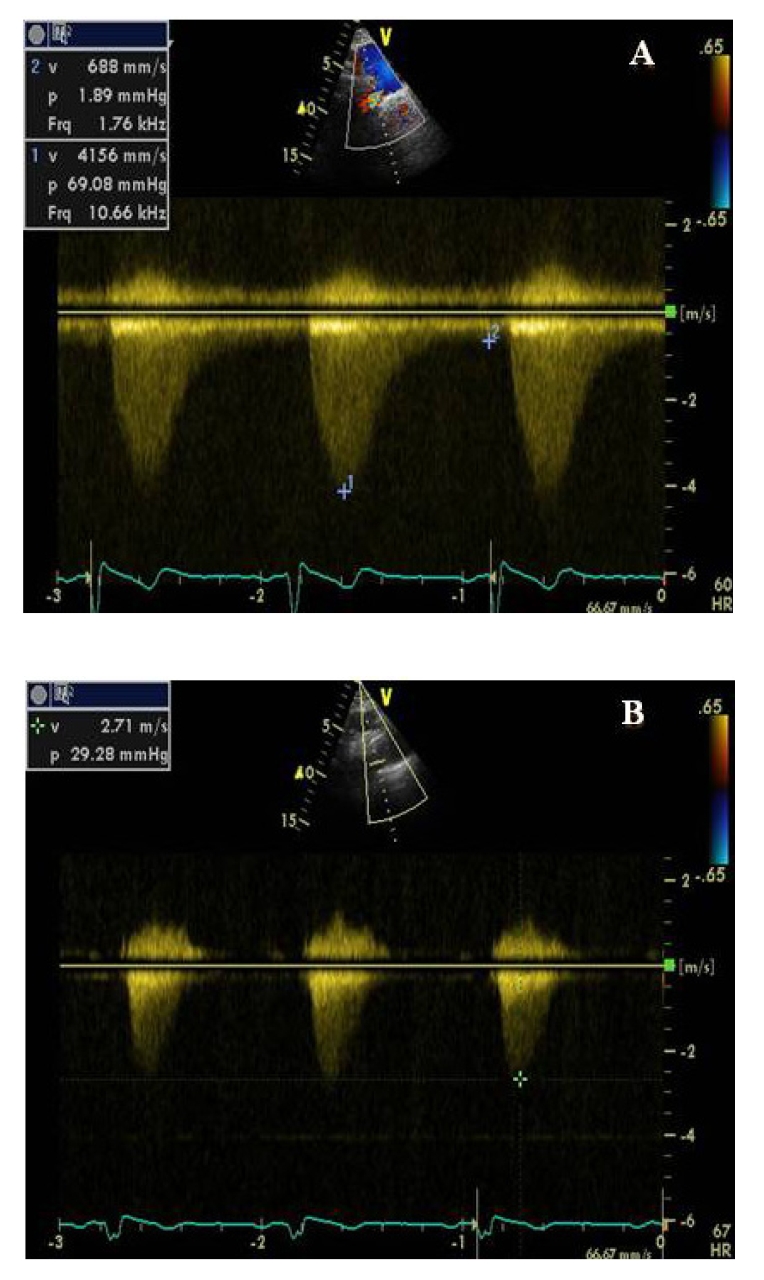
A. Continuous Doppler echocardiography showing a peak systolic gradient of 69 mmHg and a low grade antegrade diastolic flow in the descending thoracic aorta (saw tooth), highly specific for significant aortic obstruction, before stent placement. B. Reduction of the peak systolic gradient at 29 mmHg after stent placement.
